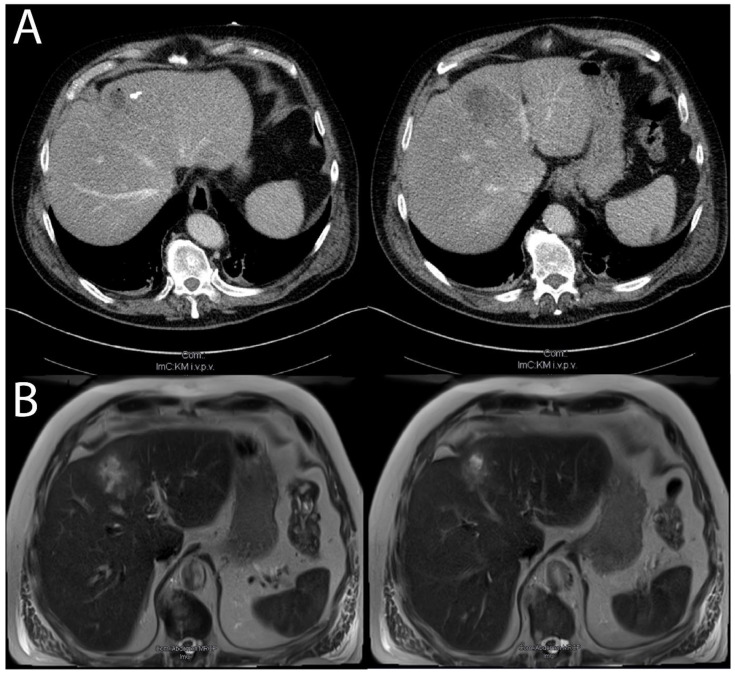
Superinfected echinococcus cyst shown in (A): CT with a contrast-enhancing agent (Accupaque) during the septic stage of disease showing a focal hypodense lesion with central air inclusions in segment IVa/IVb with calcifications on its edge (captured with Siemens SOMATOM Definition Flash, Siemens, Munich, Germany). (B): MRI, T2-weighted, of the lesion six days later after the patient had stabilized, showing a multi-chambered cyst with air inclusions and microcysts in segment IVa/IVb (captured with Siemens MAGNETOM Sola 1.5T, Siemens, Munich, Germany).
